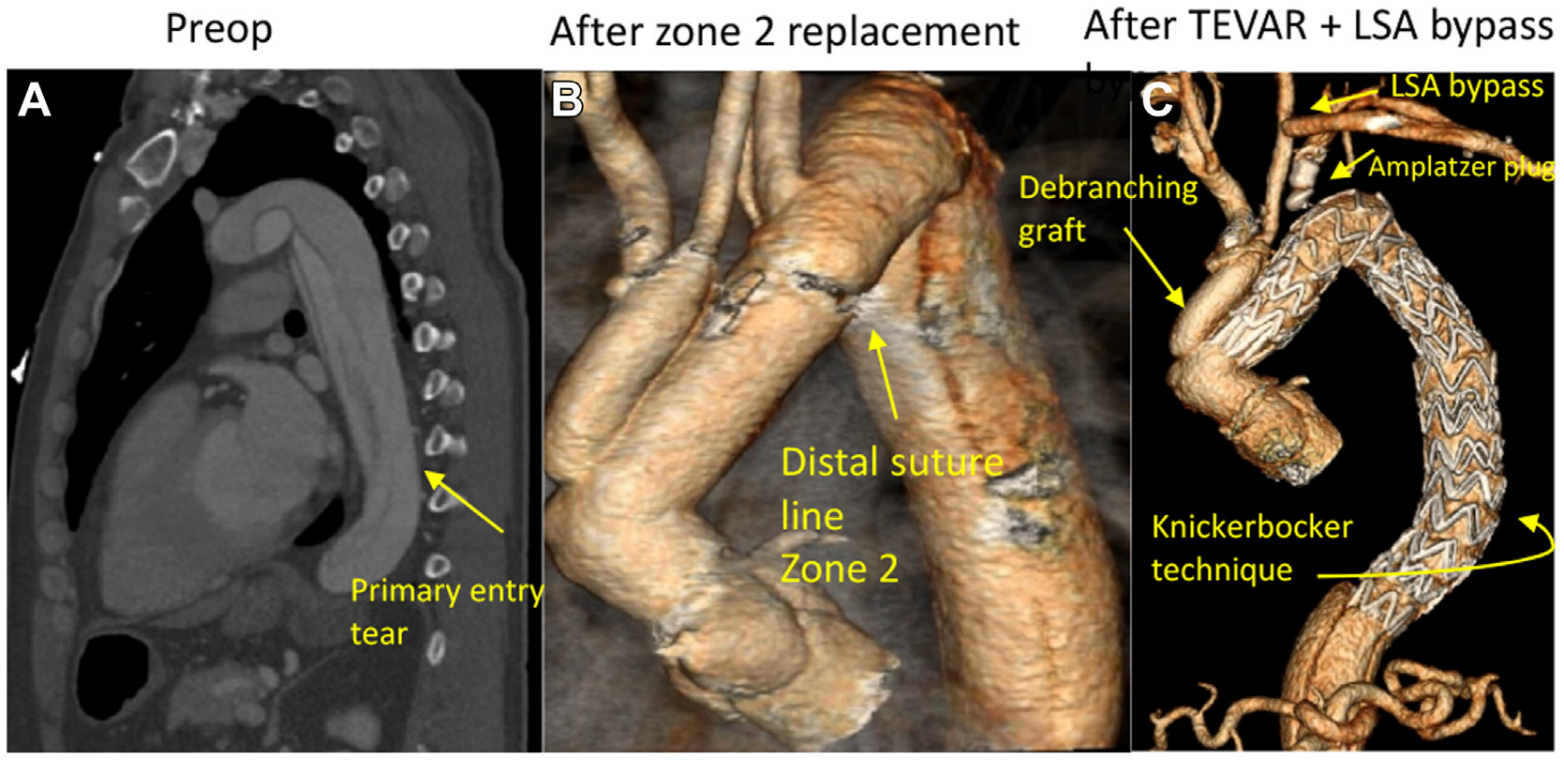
(A) Sagittal view computed tomography (CT) scan of retrograde type A dissection with the primary entry in the descending thoracic artery (arrow). (B) CT reconstruction after zone 2 arch replacement showing distal suture line just proximal to left subclavian artery (LSA). (C) CT reconstruction after staged thoracic endovascular aortic repair (TEVAR) starting just distal to the 16 mm debranching graft in the proximal ascending aorta to the celiac trunk, level of the Knickerbocker technique is depicted. Note also the LSA-left common carotid artery (LCCA) bypass and the Amplatzer plug in the proximal LSA (to prevent endoleak).
